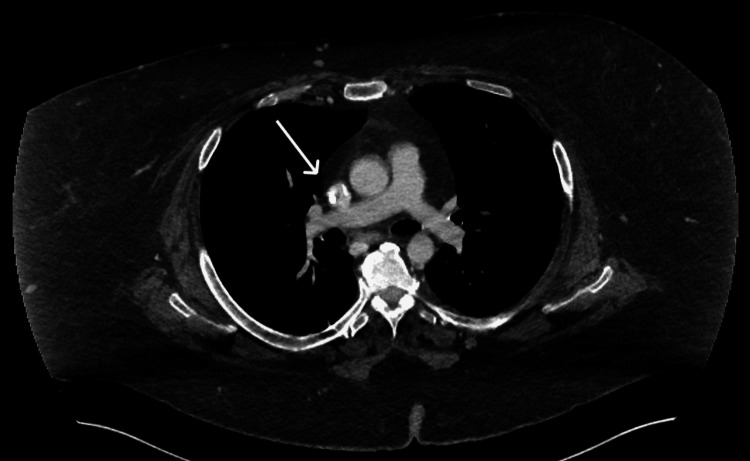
Axial contrast-enhanced CT image of the thorax demonstrating dense coronary artery calcifications marked by an arrow
